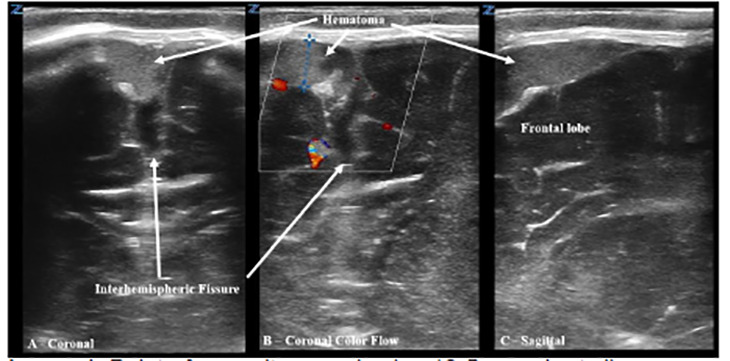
Point-of-care ultrasound using 10-5 megahertz linear probe at the anterior fontanelle demonstrating an 8-millimeter subdural hematoma in both coronal (A) and sagittal (C) orientation; and color Doppler demonstrating lack of flow (B).
